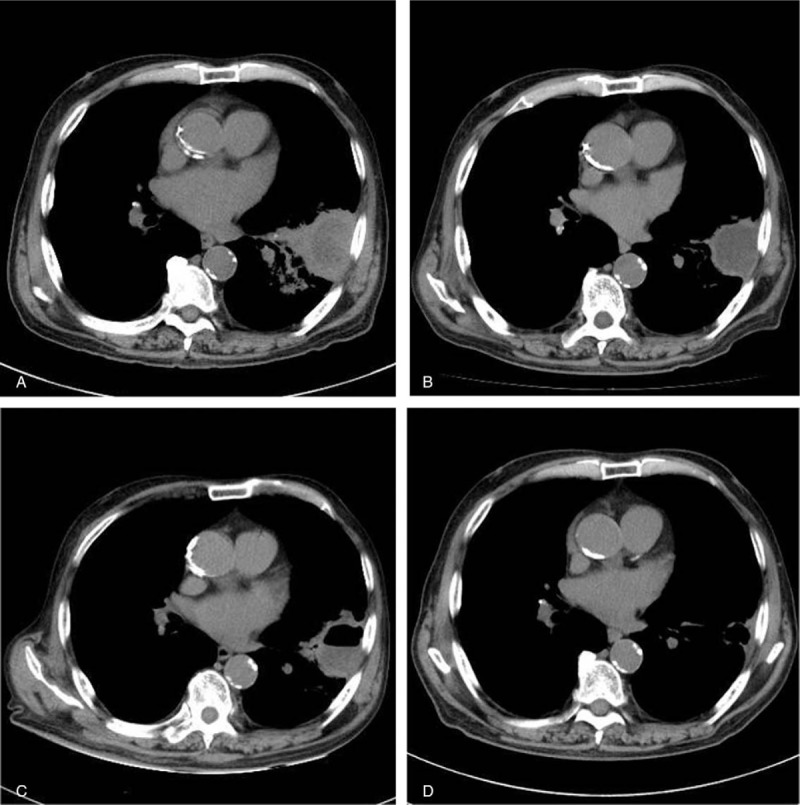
Chest CT (mediastinal window). (A) Before treatment, a 4-cm mass shadow with low-density content and a thick wall accompanied by surrounding inflammation was noted in the left lung field. (B) On hospital day 20, the wall of the mass shadow thinned and the surrounding inflammation remitted, but the low-density content remained. (C) Immediately after EBUS-GS-guided transbronchial drainage on hospital day 21, the content of the mass observed on hospital day 20 was drained, resulting in a cavity. (D) On hospital day 56, the mass had disappeared and only a slight shadow remained.
